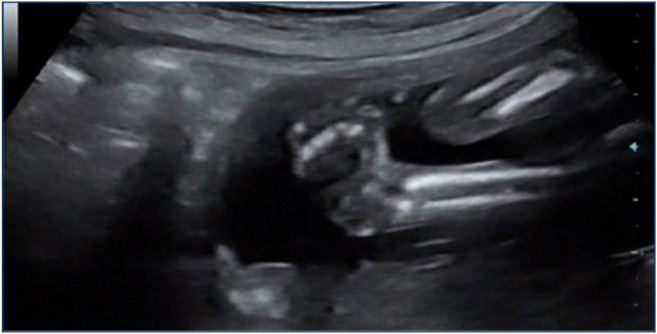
Ultrasonographic appearance of congenital talipes equinovarus: the plantar surface of the fetal foot is observed in the same sagittal plane as the tibia and fibula.
